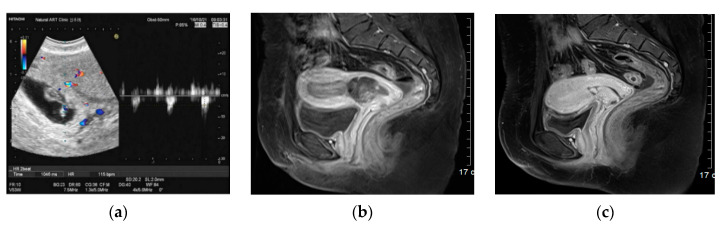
Transvaginal ultrasound Doppler image and pelvic MRI scan of cesarean section scar pregnancy with positive fetal heartbeat. (a) Transvaginal ultrasound Doppler image demonstrating FHB-positive CSSP. (b) Axial sections of pelvic MRI before the injection; initial serum β-hCG level was 43,596 mIU/mL and that at 2 h after the AE injection was 32,161 mIU/mL, demonstrating a reduction rate of 26.2%. (c) Axial sections of pelvic MRI on day 25 of the injection. Serum β-hCG level was 6.8 mIU/mL (on day 38, the level decreased to < 1.0 mIU/mL).
